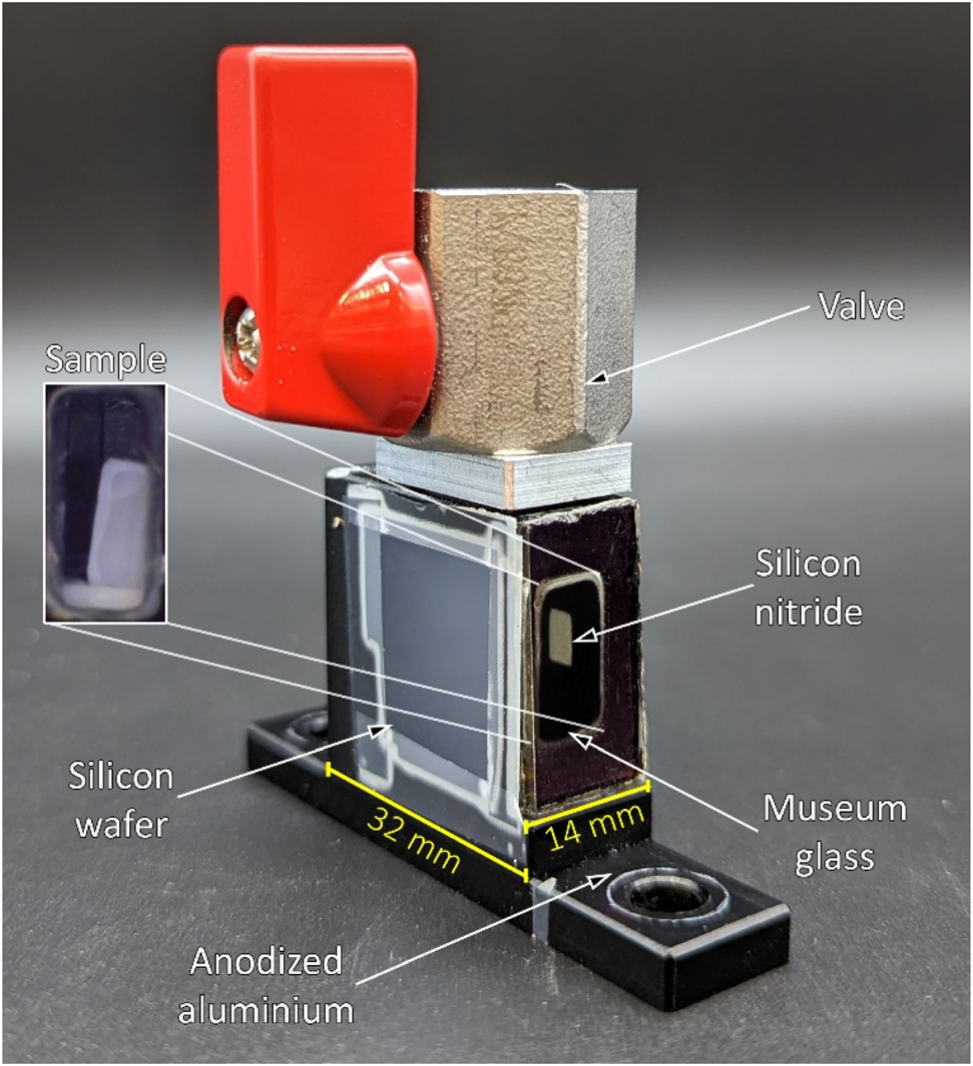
Photograph of the *in situ* measurement cell consisting of anodized aluminium, a valve on the top, a museum glass in-front, and a silicon wafer and silicon nitride window in direction of the X-ray beam. An exemplary photograph of a gel sample inside the measurement cell is shown on the left side. The scale bars (yellow) of the width and depth are given.
